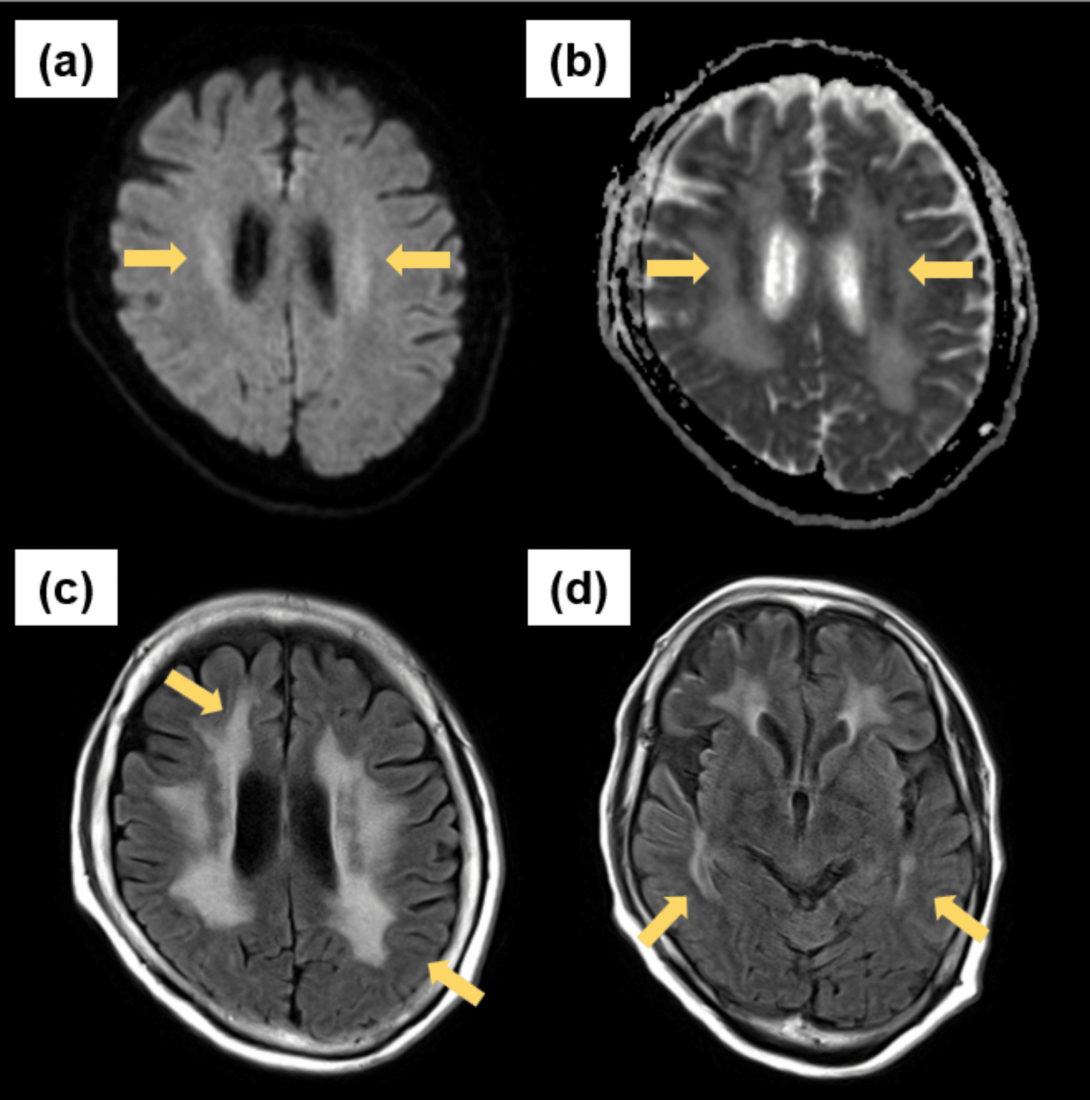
Axial MRI obtained on the 12th day (a) DWI demonstrated subtle hyperintense signals within the bilateral centrum semiovale (arrows). (b) Corresponding ADC map revealed hypointense areas, indicative of restricted diffusion (arrows). (c) Axial T2-FLAIR showed hyperintense lesions in the deep white matter (top arrow) with sparing of the subcortical U-fibers (bottom arrow). (d) Additional T2-FLAIR slice demonstrated bilateral temporal lobe hyperintensities (arrows).
